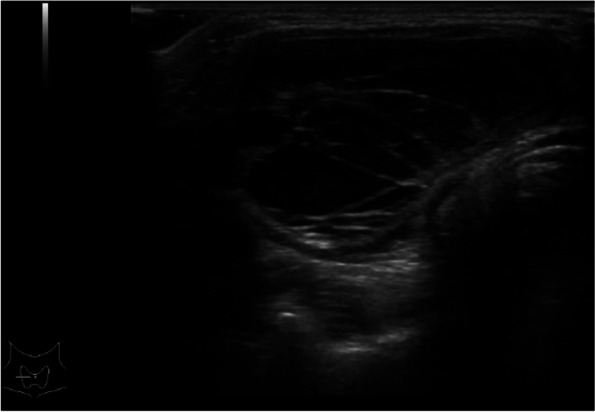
Neck ultrasonography shows a lesion with multiple cysts (4 cm × 3 cm) on the right and atrophy of the left lobe of the thyroid
